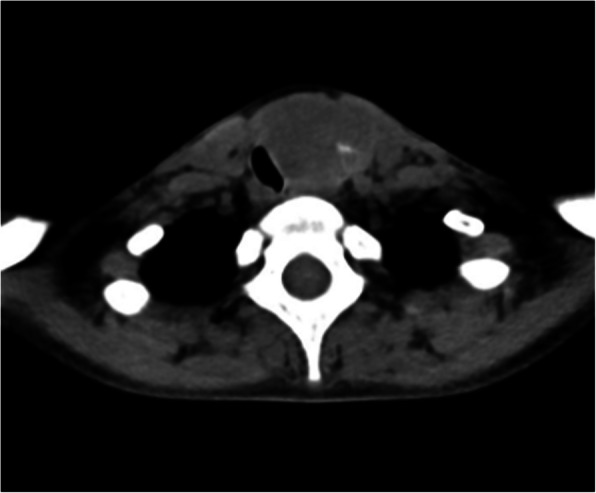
CT image showing a right tracheal deviation by a left thyroid mass
